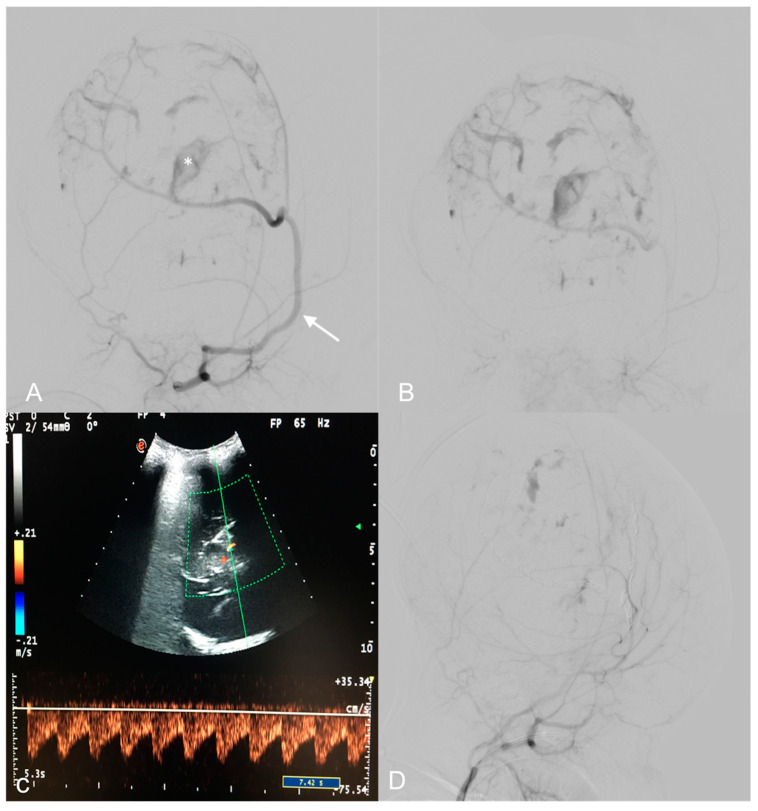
Pre-operative digital subtraction angiography (DSA) performed 1 month after birth (A,B)—Early and late acquisitions on right anterior oblique projections demonstrate arterial feeders, mainly from a hypertrophic right middle meningeal artery (arrow), minor feeders from the temporal and occipital ipsilateral arteries, and multiple intralesional blushes. A deep pseudoaneurysmatic formation (asterisk) was evident along the middle meningeal artery. US color Doppler at 7 days of life demonstrating a high, low-resistance arterial flow in an arterial feeder (C). Endovascular embolization with detachable coils and particles provided optimal devascularization of the lesion (D).
